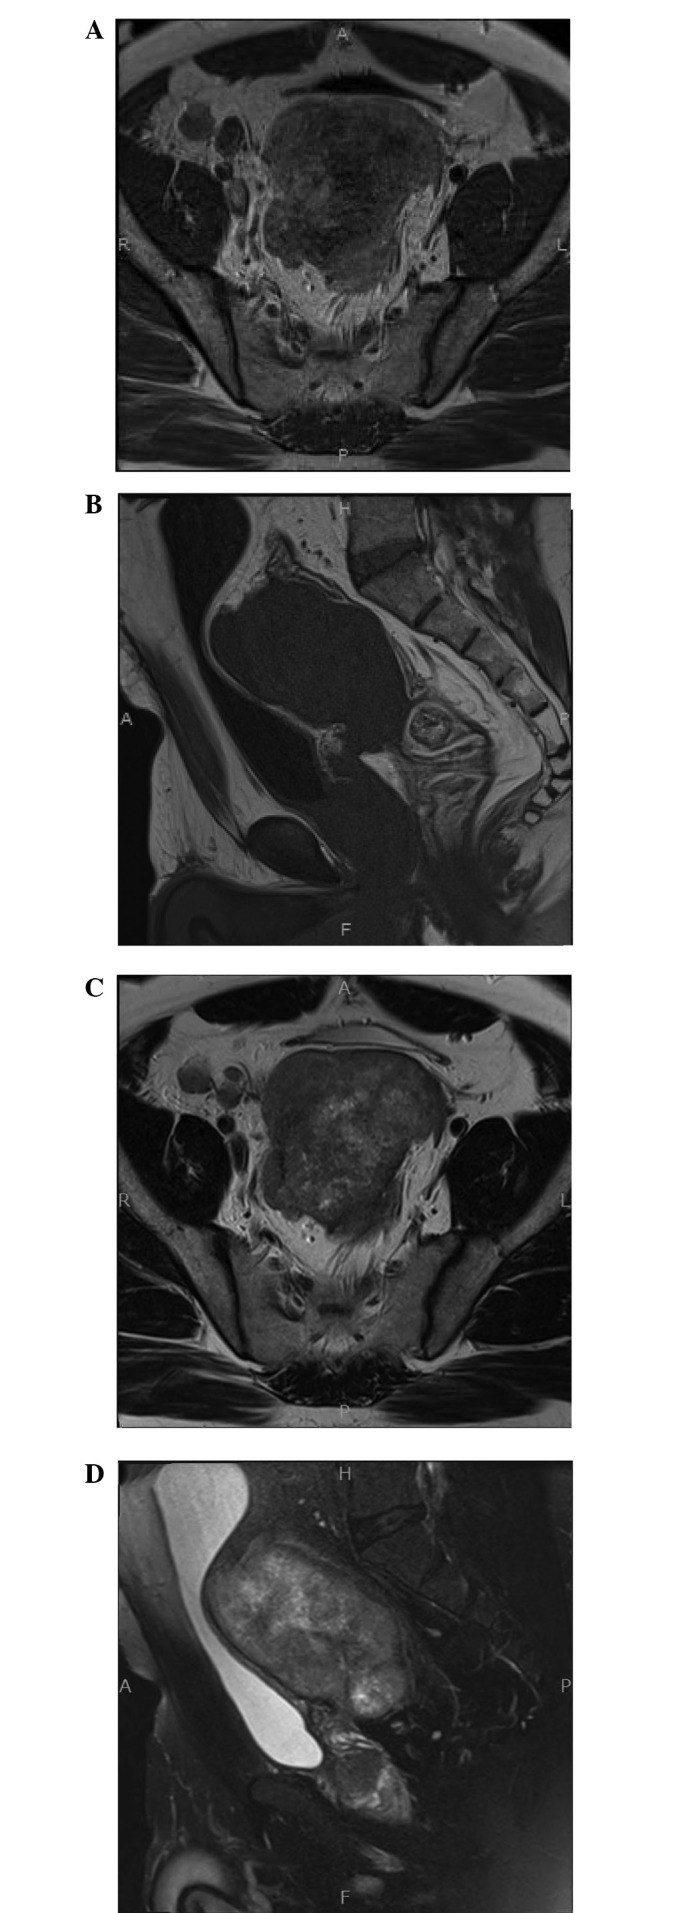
Magnetic resonance imaging. demonstrates a large lobulated mass, which compressed the bladder and rectum. (A) Axial T1-weighted. (B) Sagittal T1-weighted. (C) Axial T2-weighted. (D) Sagittal T2-weighted.
